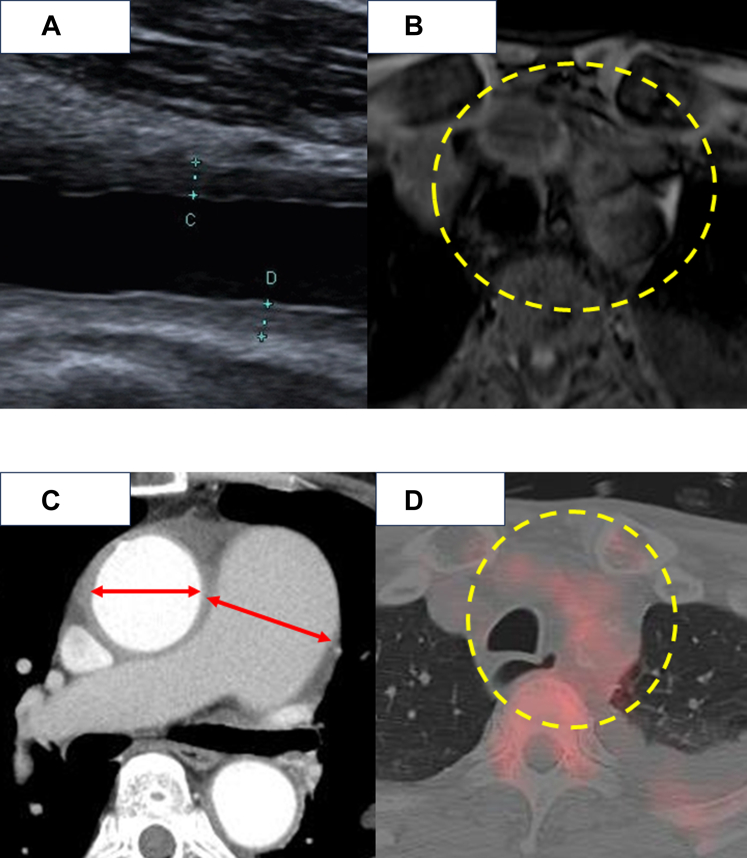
A, Carotid ultrasonography showing the characteristic “macaroni sign” in the common carotid artery, indicative of concentric wall thickening, typical of Takayasu arteritis. B, Contrast-enhanced magnetic resonance imaging demonstrating circumferential wall thickening of the cervical trifurcation arteries (highlighted by a *yellow circle*). C, Contrast-enhanced computed tomography (CT) revealing dilatation of the ascending aorta and the main pulmonary artery (*red arrows*). D, Positron emission tomography–CT showing mild radiotracer uptake in the same arterial segments (*yellow circle*), consistent with inflammatory activity.
